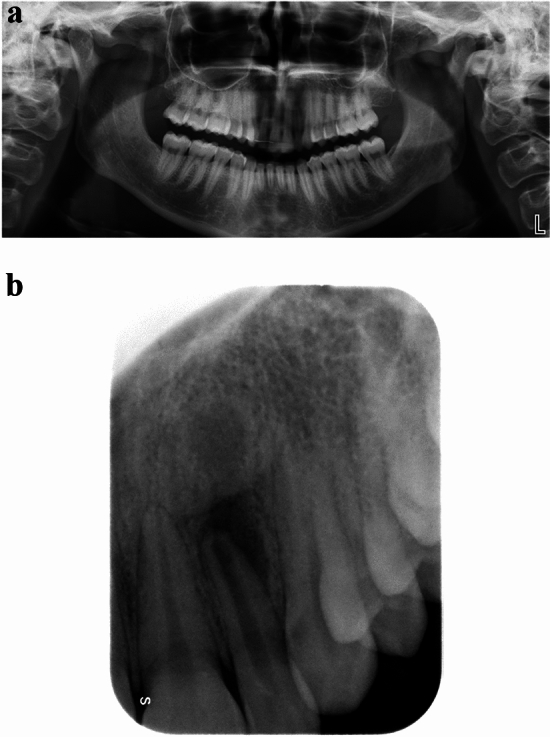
Apical periodontitis affecting the upper left lateral incisor. A In panoramic radiography, no periapical bone lesion was detected at the level of the periapex. A large area of radiolucency around incisors of both sides and especially around the root of both lateral incisors can be observed because of the overlap of the air inside the nasal cavity. B Same patient. In periapical radiography, changes in bone structure with clear mineral loss can be undoubtedly noticeable at the level of the periapex
